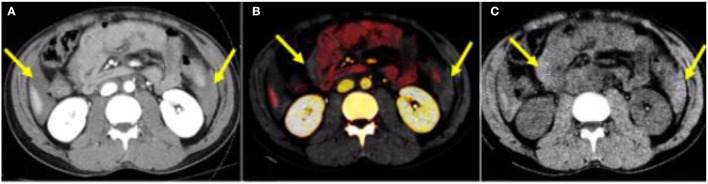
A 28-year-old male patient—after a motor vehicle accident. (A) Conventional mixed DECT image demonstrates free fluid in the abdomen (yellow arrows). (B) Iodine overlay images increase the conspicuity of free fluid by differentiating enhancing bowel from non-enhancing fluid (yellow arrows). (C) DECT VNC accentuates the hyperattenuating nature of the hemoperitoneum. DECT, dual-energy CT; VNC, virtual non-contrast.
